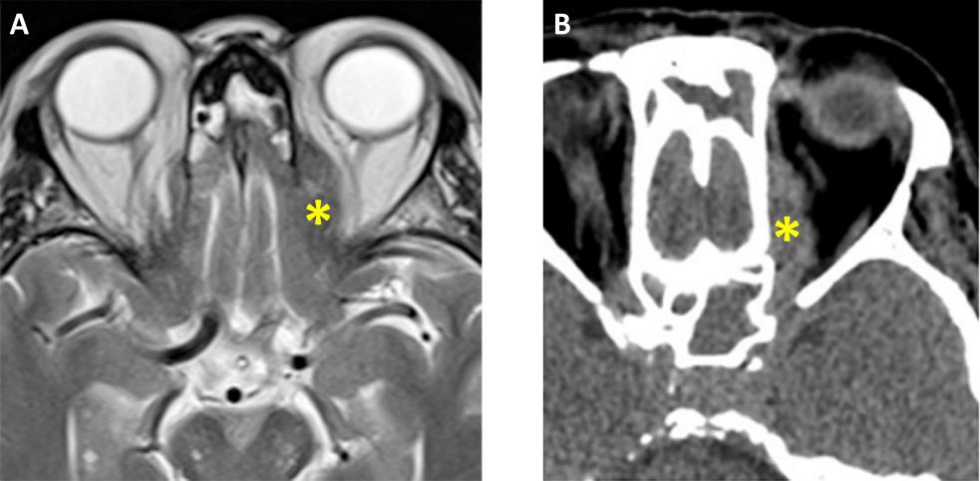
Magnetic Resonance Imaging and Computed Tomography of the Paranasal Sinuses (Axial Plane, Soft-tissue Window). A: T2-weighted MRI. B: Computed tomography. Note the better visualization of the left orbit invasion (asterisks) through the medial wall by MRI (A), as opposed to CT (B).
